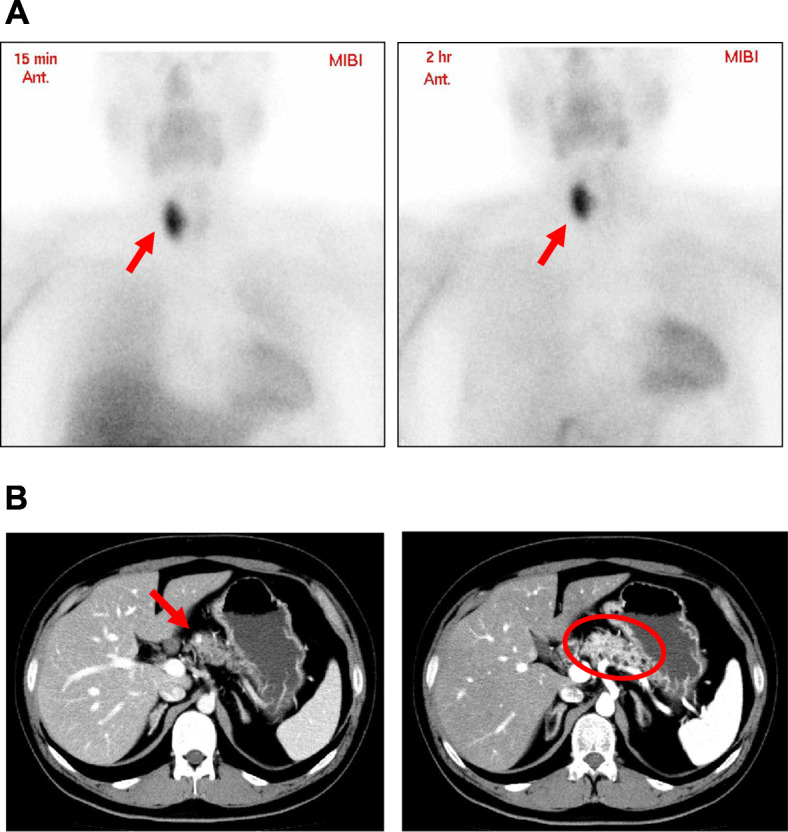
(A) In ^99m^Tc MIBI scintigraphy, MIBC accumulation was observed at 2 h as well as at 15 min in neck lesion which was thought to be parathyroid lesion (arrows in both panels). (B) Contrast-enhanced abdominal CT. Small tumor (diameter: 5 mm) was observed in the pancreatic head (an arrow in left panel). Multiple fat density areas were observed in the whole pancreas (a circle in right panel)
